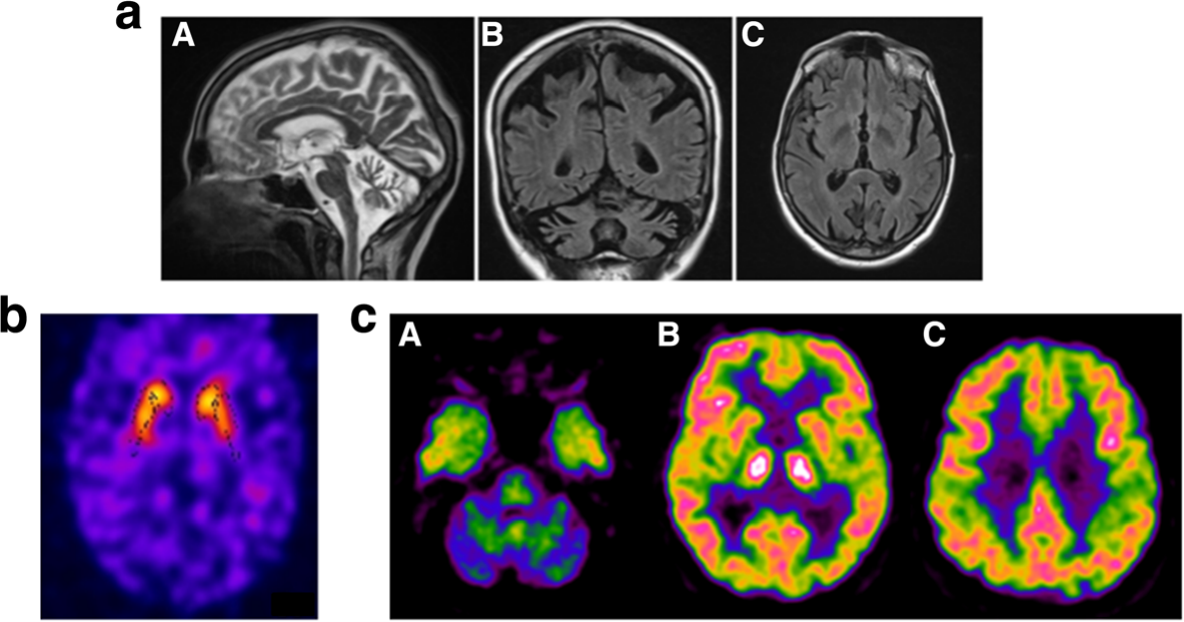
a Sagittal brain MRI (T2-weighted) disclosing gross and diffuse atrophy involving the cerebellum (A). Coronal brain MRI (T1-weighted) showing symmetrical atrophy of cerebellum and parietal cortex (B). Axial brain MRI (FLAIR) showing preservation of basal ganglia (C). b DAT-SPECT with I-123 ioflupane showed globally normal standardized binding ratios with only mild reduction of the putamen-to-caudate ratio in the left hemisphere (0.64). Semi quantification was achieved through the Basal ganglia software V2 (Calvini et al., [15]). c 18F- FDG PET showed severely decreased uptake in both cerebellar hemispheres (A) and moderate decreased uptake in the two putamen, mainly in the left hemisphere (B). No cortical uptake deficit was found (B, C). Hot colors (white, red, yellow) report higher uptake than cold colors (green, blue)
